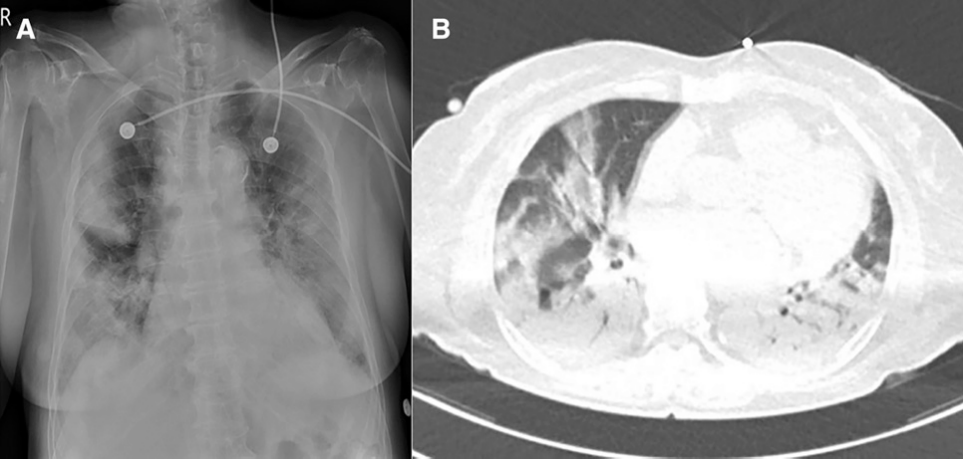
Chest radiograph. (A) Bilateral peripheral and lower lung zone opacities of atypical pneumonia. Chest computed tomography. (B) Multiple ground-glass opacities in the periphery and lower lobes, consistent with atypical pneumonia with diffuse alveolar damage.
